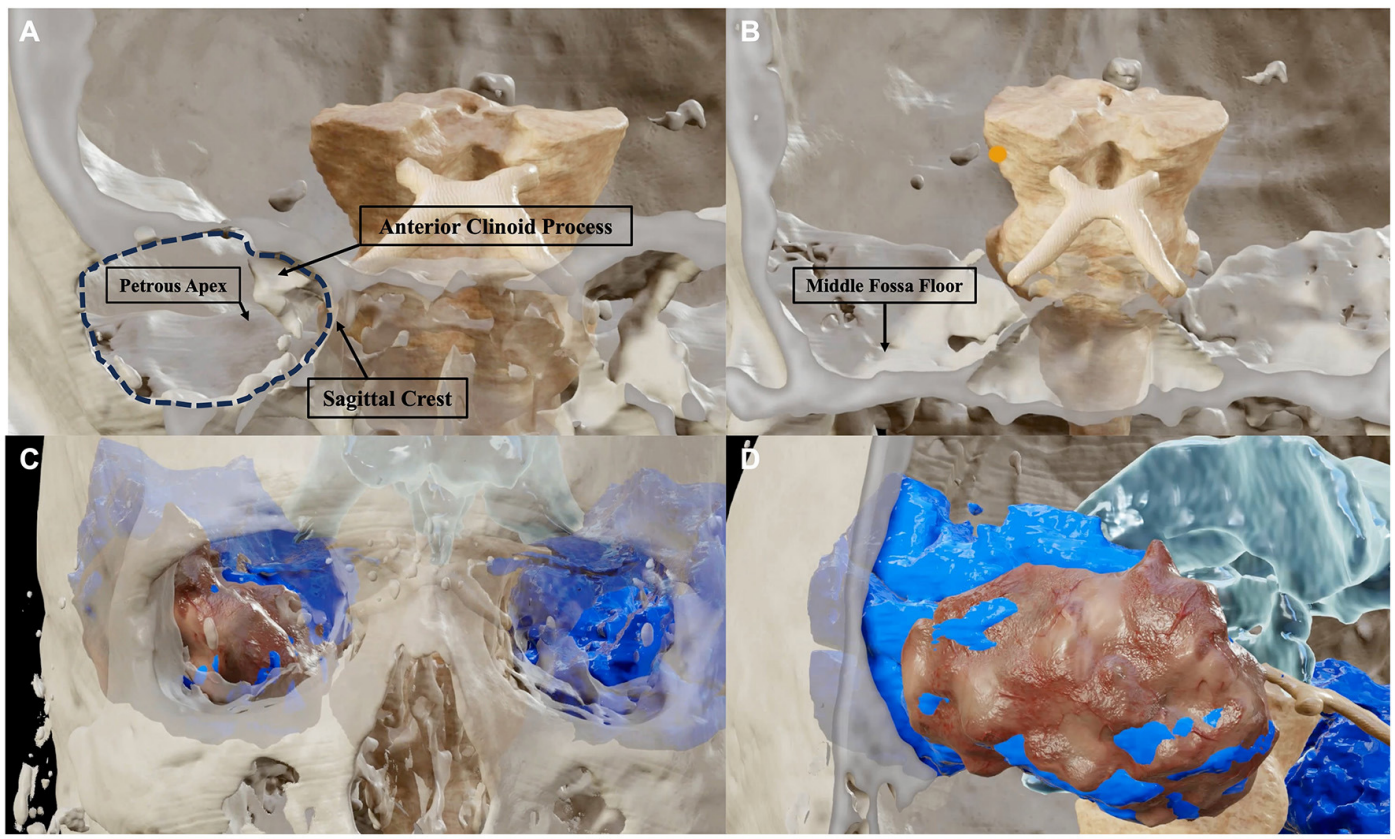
BrainLab^®^ reconstructions of post-dissection CT and MRI scans highlighting the relationship between the amount of bone removal (A, B) with the amount of resection of the temporal lobe (C, D). The bone work of the approach is limited to an anterior sphenoidal craniotomy (dark dotted area) which provides access to the temporal pole. Three of the four bony pillars of the approach, namely, the sagittal crest, the anterior clinoid process, and the petrous apex are left intact (A). The middle cranial fossa floor, which represents the fourth bony pillar, must be flattened to provide adequate maneuverability for the instruments which must work in parallel to the basal surface of the temporal lobe (B). The amount of removal of the temporal lobe (brown reconstruction) in relation with the total of the temporal lobe (blue reconstruction) is shown in an anterior (C) and antero-lateral (D) perspective.
